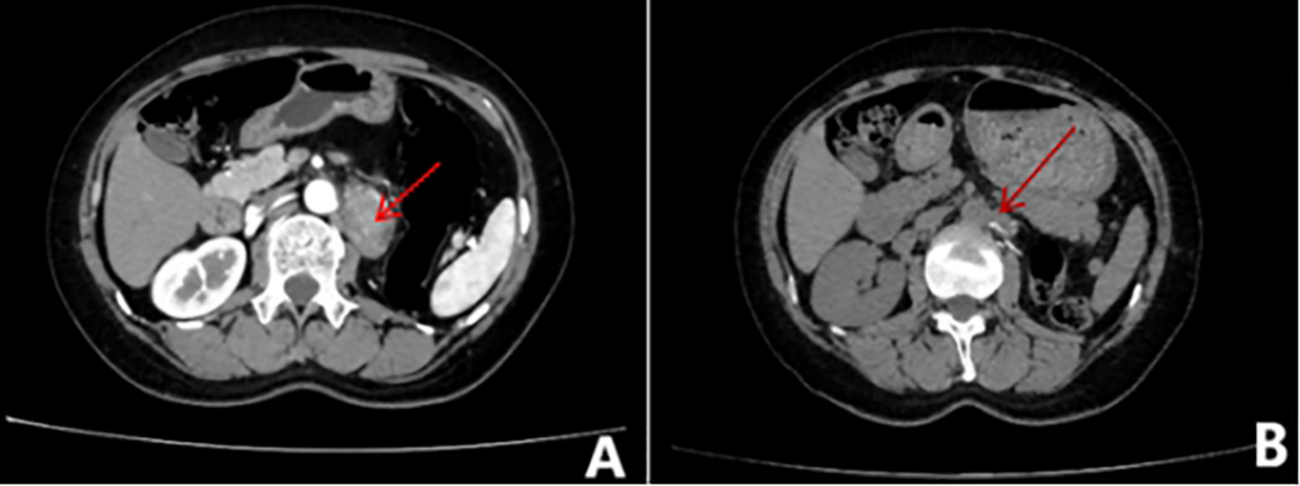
Whole abdominal contrast-enhanced CT scan. (A) Before treatment, metastases were visible in the medial left adrenal gland, left paraabdominal aorta, left peritoneum, and omental area. (B) After treatment, Metastases can be seen in the parietal peritoneum, omentum, and retroperitoneal space of the left mid-abdominal cavity and on the left side of the abdominal aorta.
